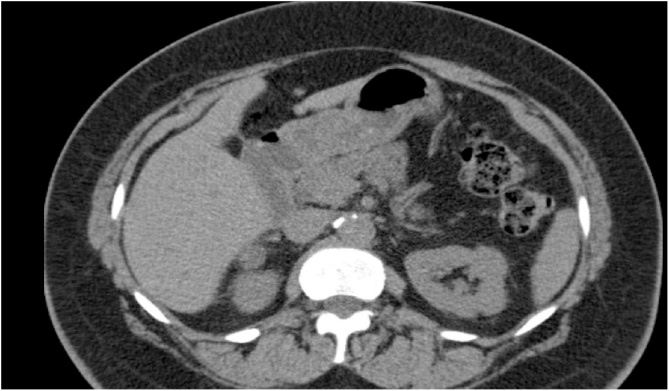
Non-contrast CT showing a 1.5 cm nodule involving the adrenal gland with a Hounsfield unit density of 14. On MRI, this was interpreted as a benign adenoma.
